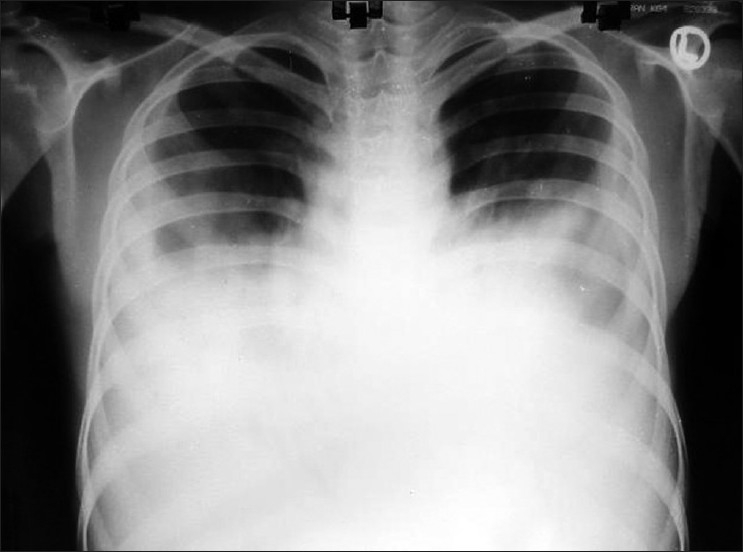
Chest X-ray revealed bilateral pleural effusion
